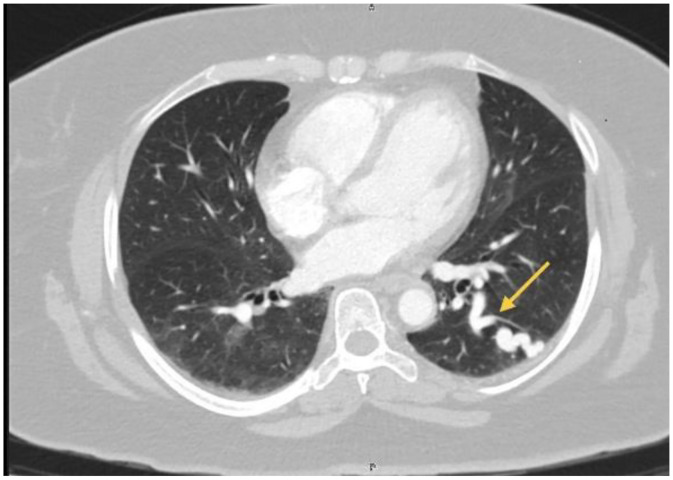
Chest CT scan with IV contrast, axial view, in the left lung, posterior basal region, shows a serpiginous structure of high attenuation following the course of a vessel (yellow arrow) with both arterial and venous dilation, consistent with a pulmonary arteriovenous malformation (arteriovenous fistula). A prominent arterial afferent vessel and a small aneurysmal sac with its corresponding efferent vein draining into the pulmonary venous circulation are observed.
